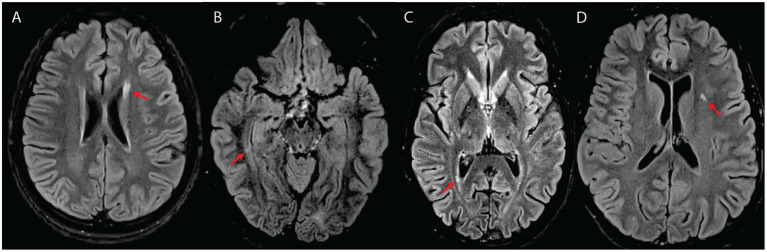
White matter abnormalities suggestive of an inflammatory process (A–C) or classified as intermediate (D) in four subjects with WFS. All images are axial T2-FLAIR. (A) Elongated lesion located at the level of the anterior horn of the left lateral ventricle (subject #7). (B) Small temporal periventricular lesion perpendicular to the right ventricular wall (subject #7). (C) Small periventricular lesion perpendicular to the right ventricular wall (subject #10). (D) Left insular deep white matter lesion classified as intermediate between suspicious for inflammatory process and typical for WFS (subject #8).
